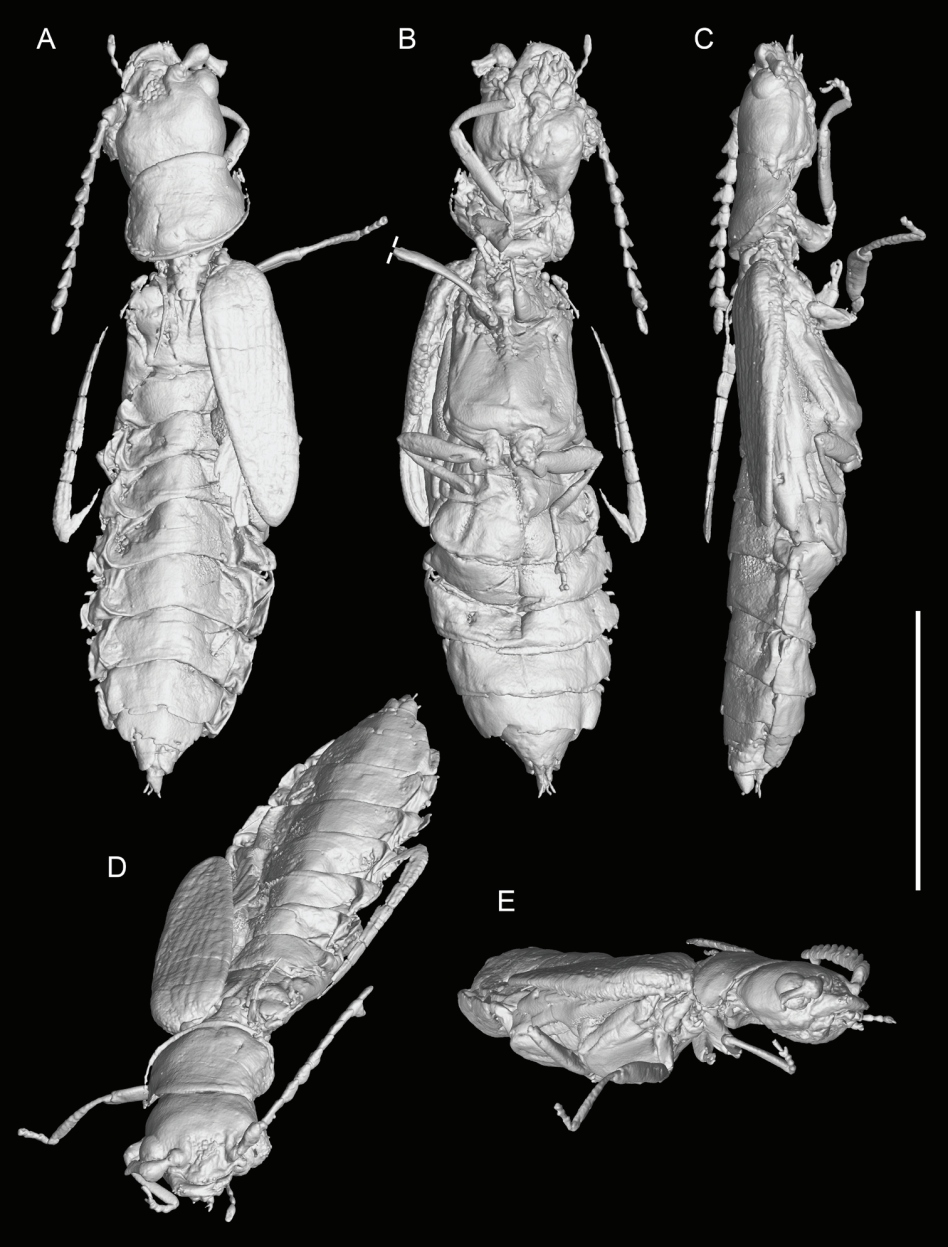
X-ray microtomographic reconstruction of *Nothotytthonyxserratus* Li, Biffi, Kundrata & Cai sp. nov., holotype, NIGP179427 A dorsal view B ventral view C lateral view D anterodorsal view E anterolateral view. Scale bar: 2 mm.
